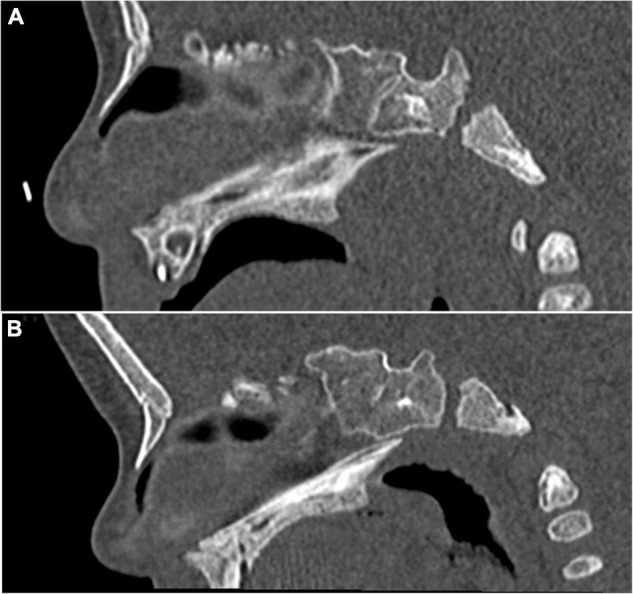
Head CT images for palate comparison. (A) Deceased 5-month-old male. Anteroposterior incurvation of the palate can be noticed, with a high palatine arch. The palate was considered to be ogival. (B) Living 5-month-old male. CT was performed to eliminate intracranial blood. The palate is almost flat, without anteroposterior incurvation, and was considered to be non-ogival.
